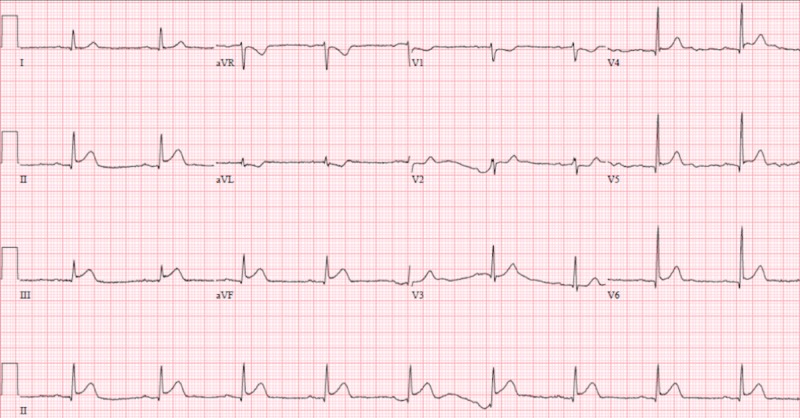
12-lead echocardiogram demonstrating ST-elevation in inferolateral leads (II, III, aVF, V5, V6)
